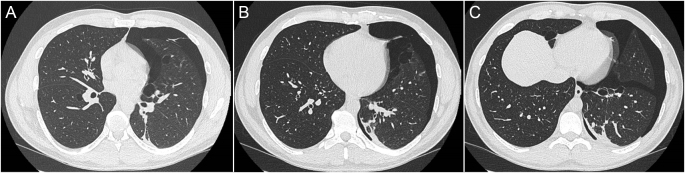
Preoperative CT imaging demonstrating (A) left upper lobe bullae; (B) left upper and right lower lobe bullae; (C) left lower lobe bullae.
